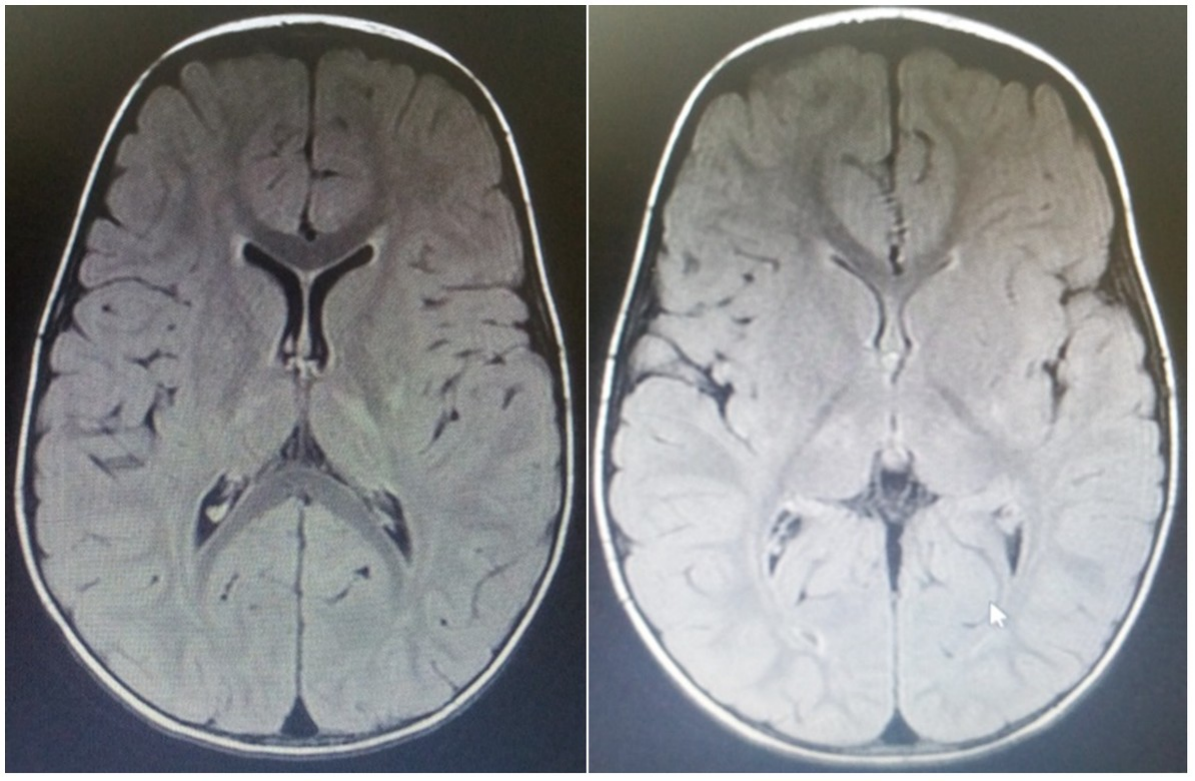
Case 19: MRI of a 3 year old male patient with unspecified form. MRI images of a 3 year old male child delivered at term who did not cry immediately after birth. Axial T2 FLAIR MR images showing bilateral basal ganglia and thalamic hyperintensities.
